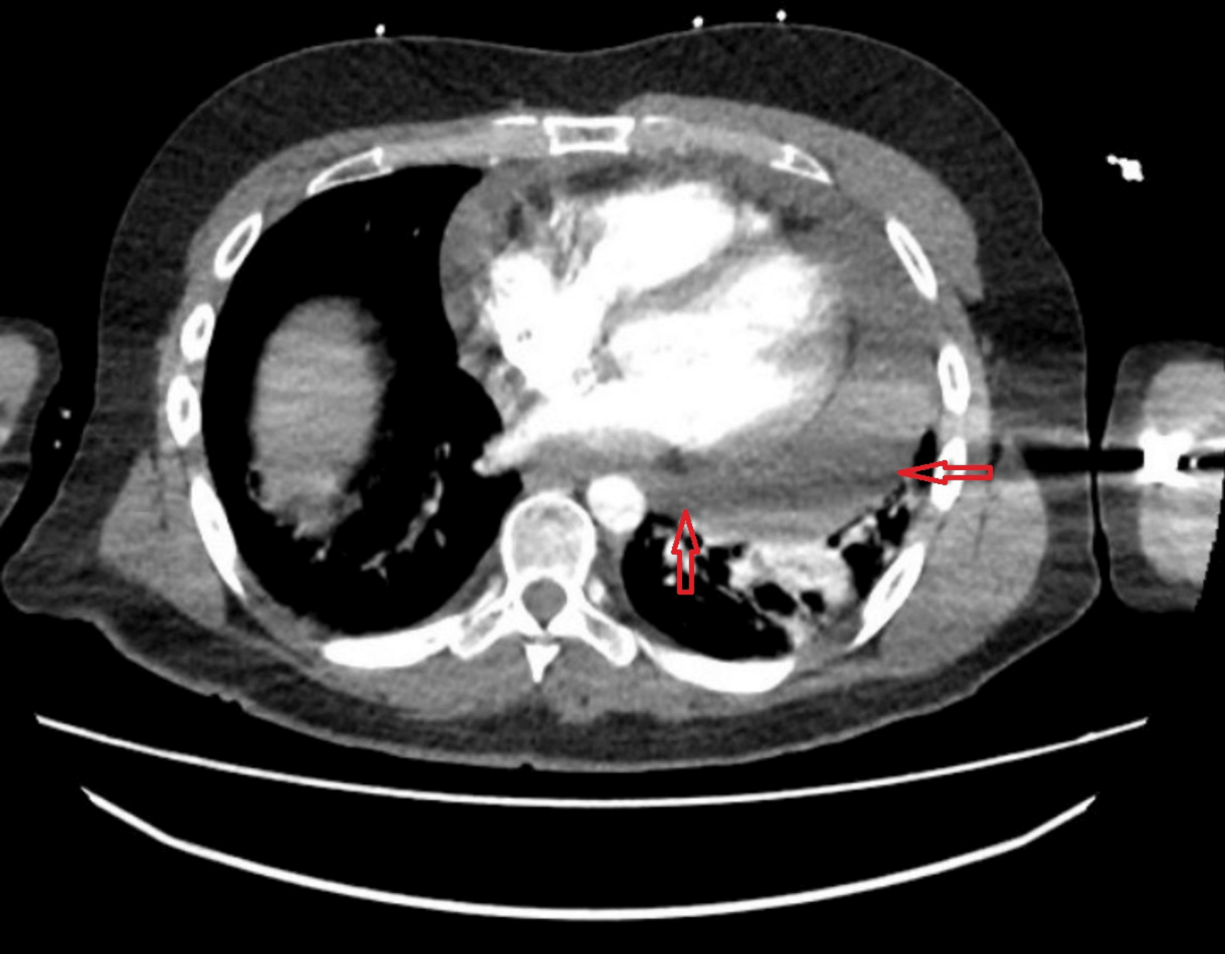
CT angiography chest showing a large pericardial effusion. CT: Computed tomography
